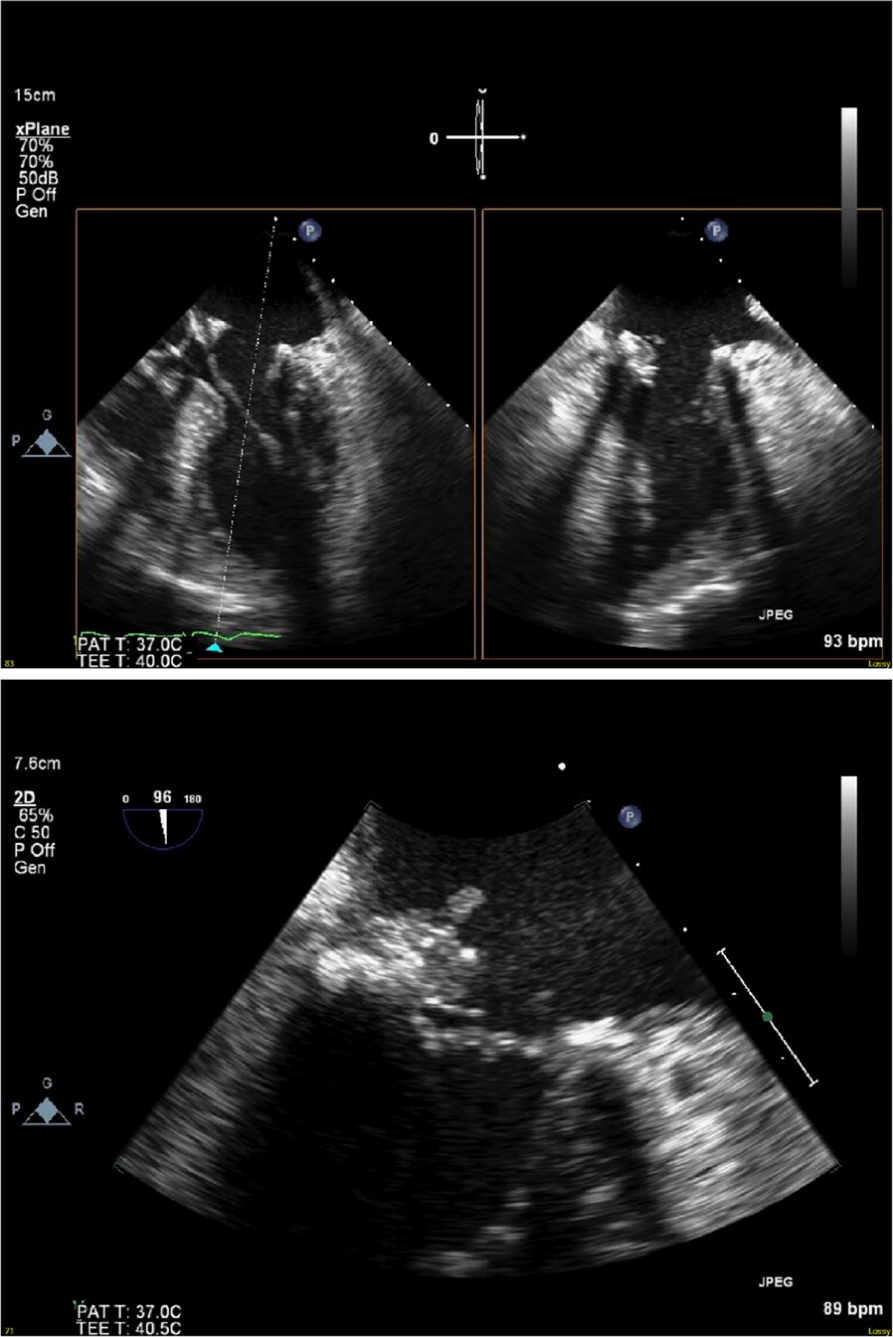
Trans-esophageal echocardiogram showing mitral valve vegetation in the mid-esophageal plane
